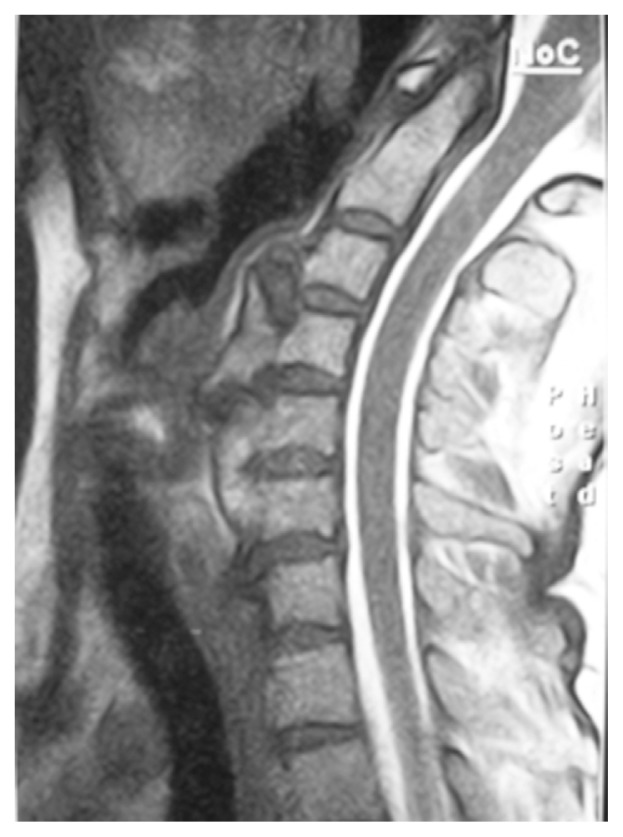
Sagittal T2-w MRI demonstrating excessive anterior osteophyte formations and ossificated anterior longitudinal ligament compressing on the esophagus at multiple levels.
